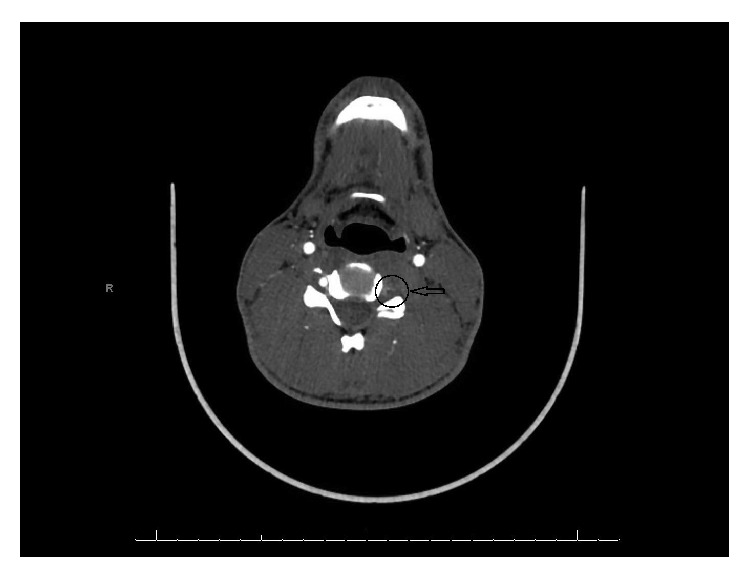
CT Angiogram showing hyperattenuating crescent shaped mural thickening of left vertebral artery (marked by black circle and arrow).
